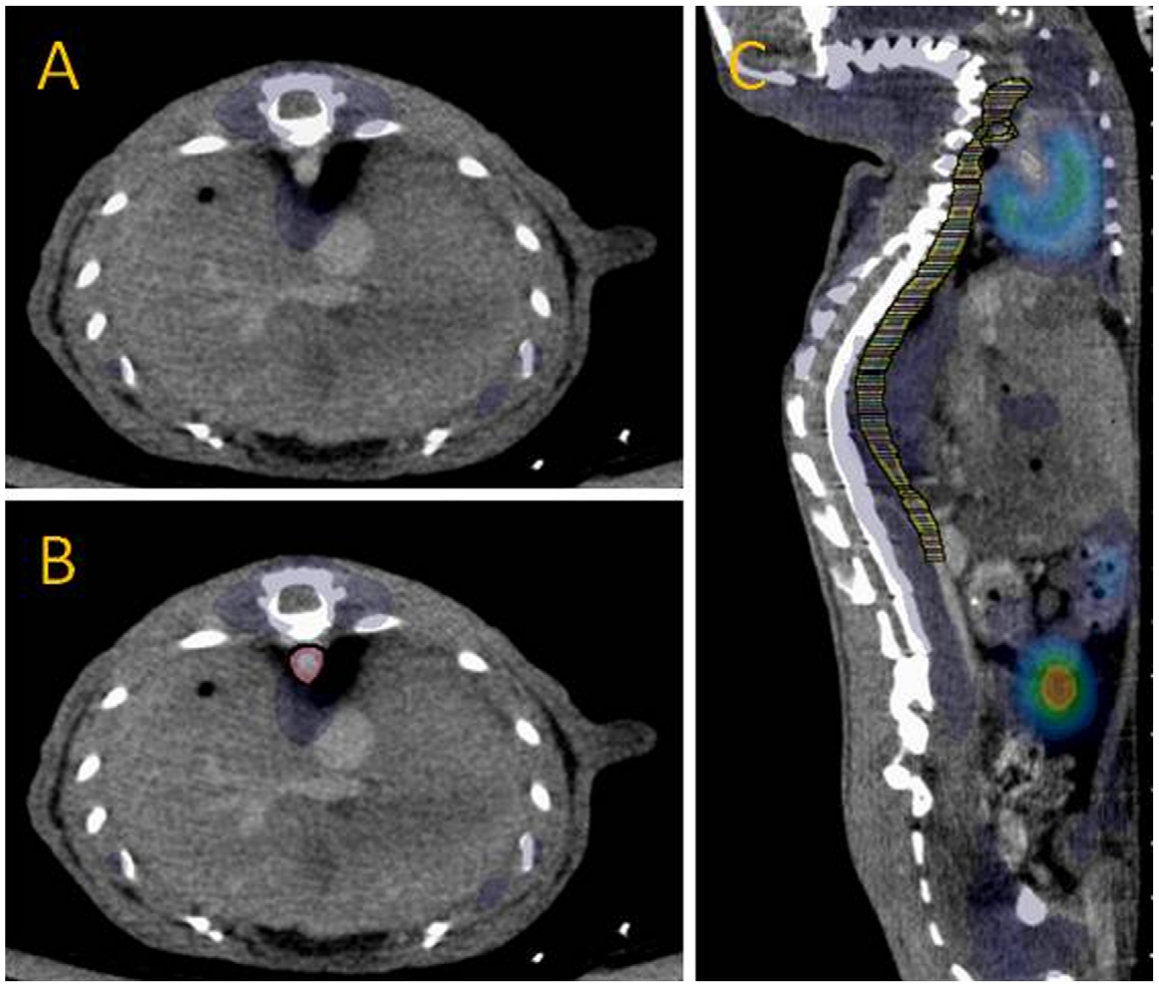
Selection of ROIs. A Fused PET/CT image, axial view. B Fused PET/CT image with ROI drawn, axial view. C Fused PET/CT image with the ROIs after ROI interpolation, sagittal view. Most, but not the entire aorta is visible.
